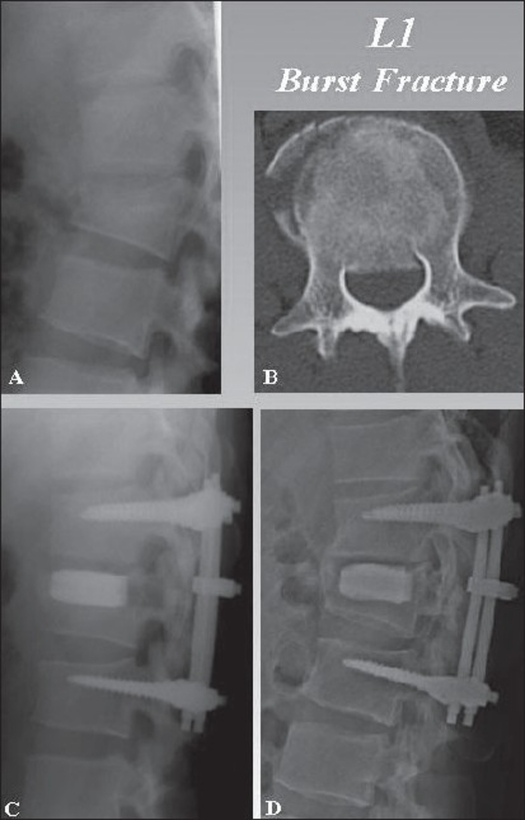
Lateral X-ray of dorsal lumbar spine (A) shows burst fracture of L1. Axial CT (B) of L1 vertebra shows burst fracture with cord compression. Lateral X-ray of immediate postoperative (C) and final followup (D) show well placed transpedicle body augmenter with restoration of anterior body height and kyphosis
